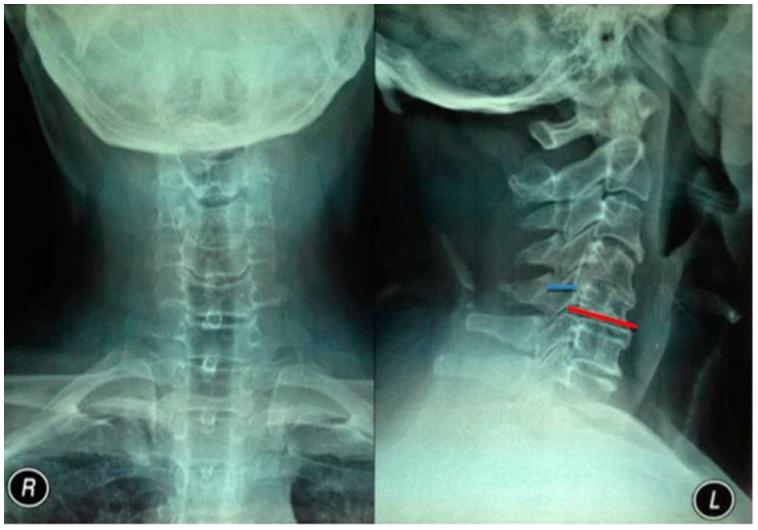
Preoperative cervical spine radiography image from (A) the anteroposterior view and (B) the lateral view.
